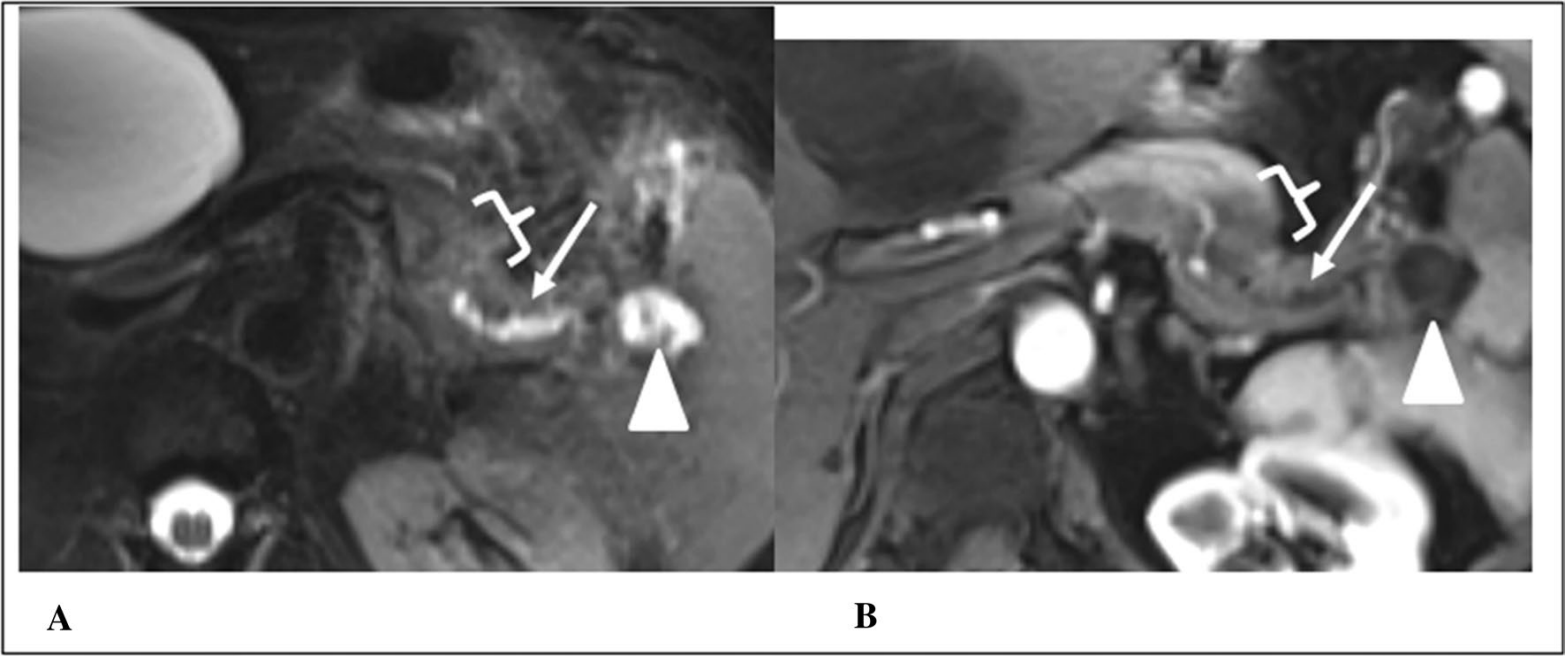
RADPEER 3 score on MRI—missed finding and satisfaction of search. A case of a 63-year old female that underwent an MRI because of a presumed episode of pancreatitis. Axial T2w fat saturated (A) and axial T1w fat saturated arterial phase image (B) from an outside institution demonstrate pancreatic duct dilation (arrows) and disruption terminating at a 25 mm hypoenhancing tail mass (brackets) with small pseudocyst (arrowhead) in the splenic hilum. The initial interpretation described the pseudocyst and multiple liver cysts; an example of missed finding and satisfaction of search. Both expert readers independently identified the mass and secondary findings with high confidence. The patient presented with recurrent symptoms 8 months later and abdominal imaging revealed a pancreatic tail mass
